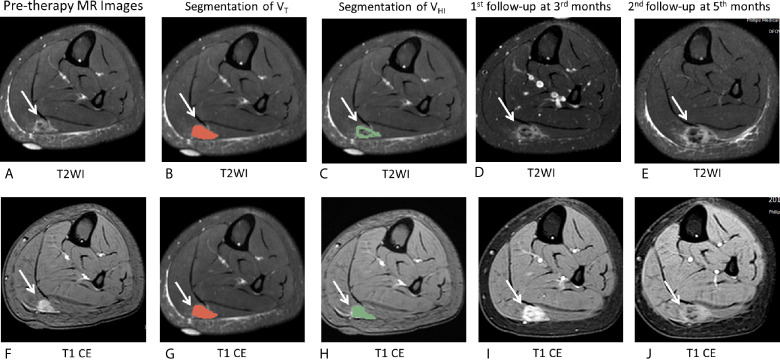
MR images of the representative case. (A–J), A 16-year-old female patient with DF of right calf muscle with imatinib treatment (arrows). Pre-therapy MR images refer to examination before imatinib treatment. Pre-therapy (A, F) diameter, 20.0 mm; On T2WI, V_T_ = 6,242 mm^3^, V_HI_ = 6,242 mm^3^, HI = 100% (grade = 3); On T1 CE, V_T_ = 6,357 mm^3^, V_HI_ = 6,357 mm^3^, HI = 100% (grade = 3). Segmentation of V_T_ (B, G, red zone) and segmentation of V_HI_ (C, H, green zone). 1^st^ follow-up at 3 months after imatinib treatment (D, I) Diameter, 23.5 mm indicates a 17.5% increase in tumor size as well as a decrease in T2 and T1 CE signal intensity. The tumor response is SD, according to RECIST1.1. Second follow-up at 6 months since imatinib treatment (E, J) diameter, 48.0 mm shows a 240% increase in tumor size as well as a decrease in T2 and T1 CE signal intensity. The tumor response assessment is PD, according to RECIST1.1. After the second follow-up examination, this patient changed treatment strategy to chemotherapy.
